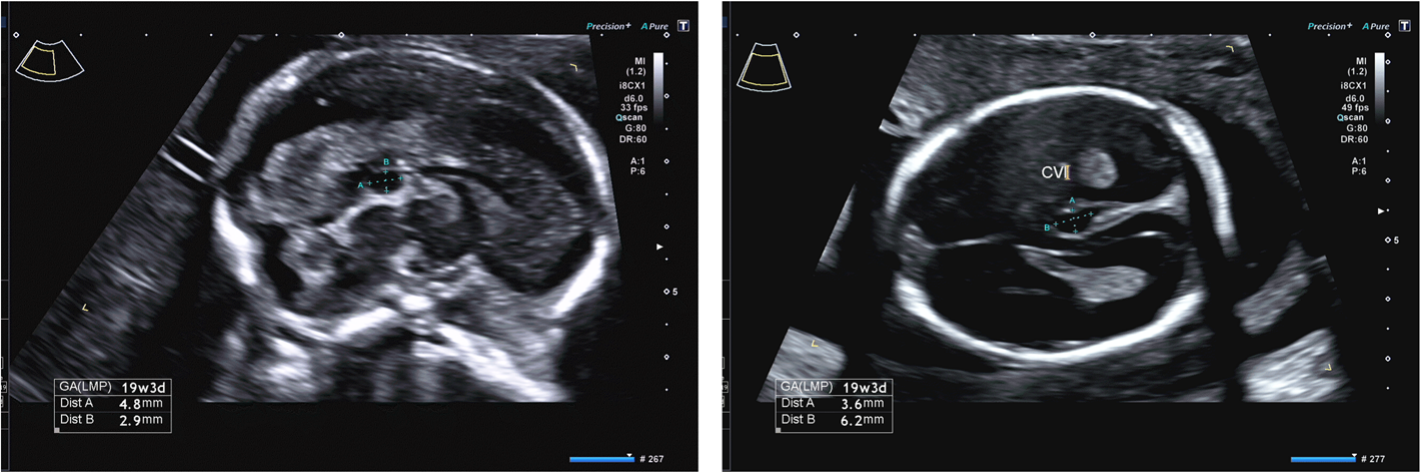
Ultrasound images  of the cyst in both foetuses. Legend: Sagittal view of the cyst in the foetus a (left), axial view of the cyst in the foetus b (right). The cyst size can be seen in the bottom left corner of both images
